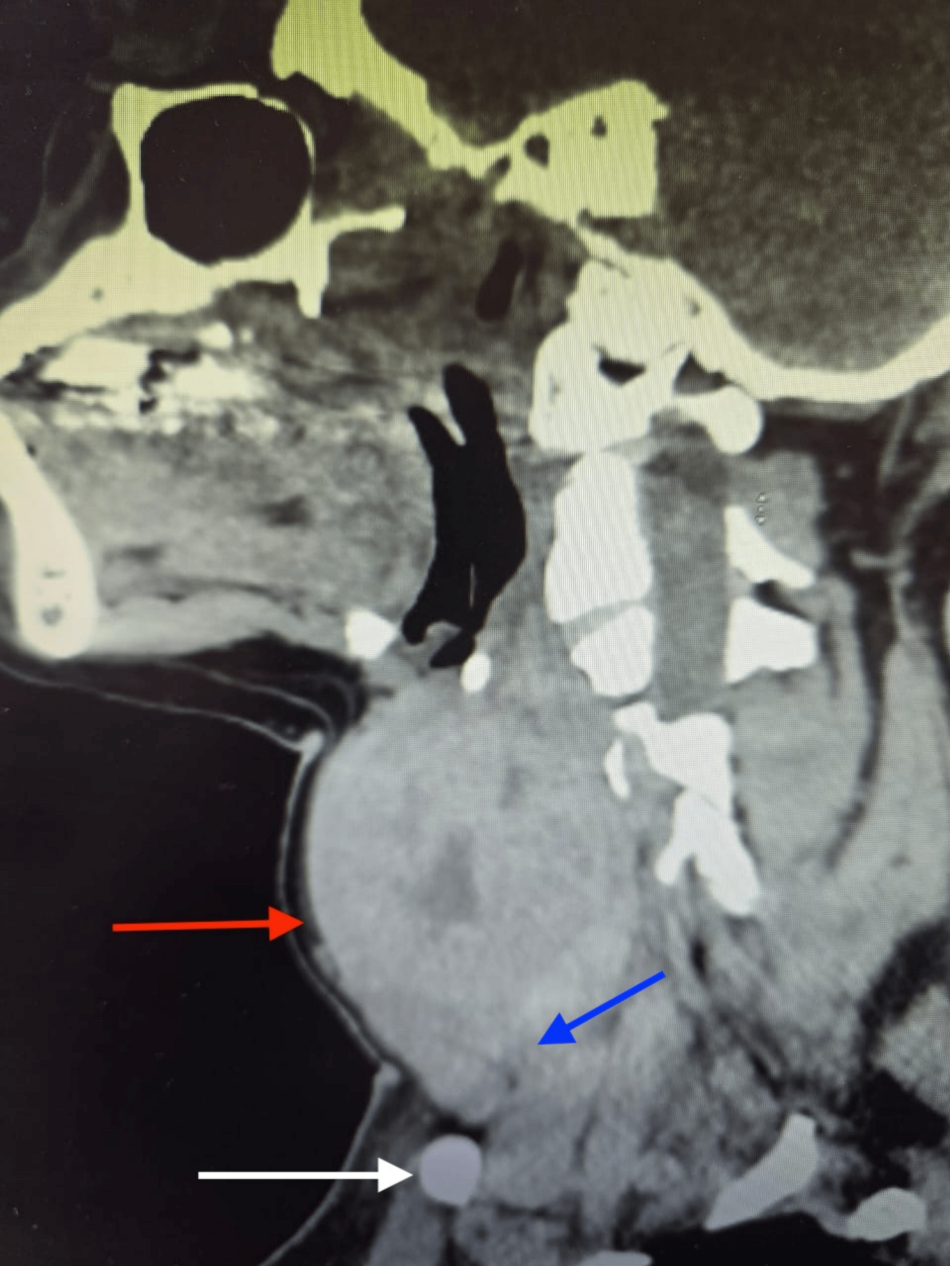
Sagittal non-contrast CT image of the neck demonstrating a markedly enlarged thyroid gland. The giant thyroid enlargement is identified occupying the lower cervical region (red arrow). Inferior extension of the thyroid gland into the retrosternal space is demonstrated, consistent with retrosternal extension (blue arrow). The clavicle is visualized as a bony landmark anteriorly (white arrow).
